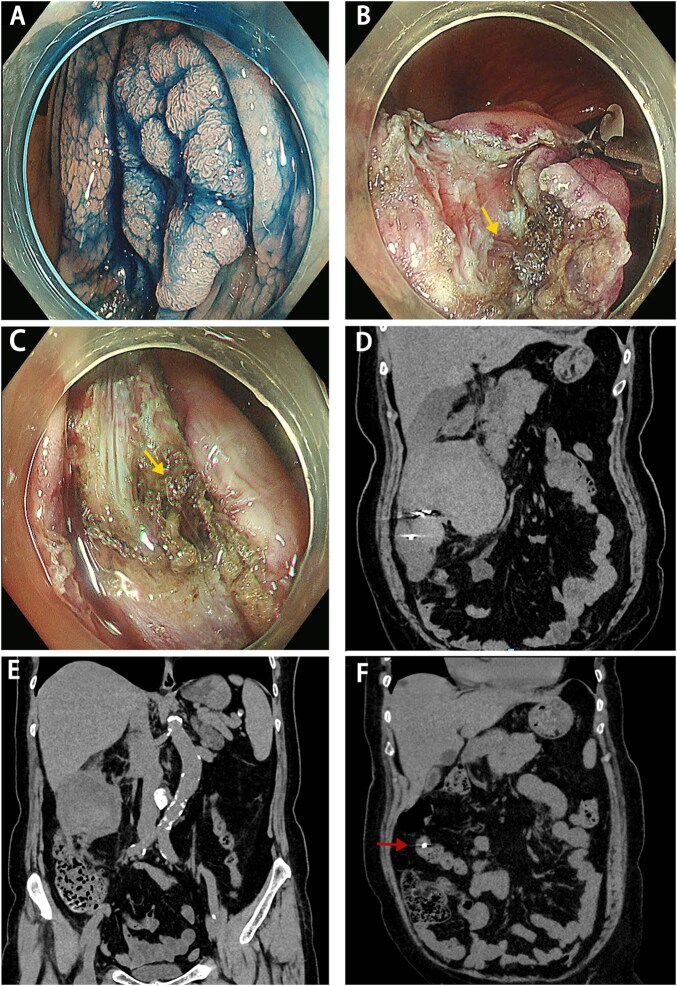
The clinical data of the case with paracolic gutter hematoma after colonic endoscopic submucosal dissection. (A) Indigo carmine chromoendoscopy demonstrated a lateral spreading tumor ∼3 cm in size in the ascending colon. (B) Several perforating vessels originating from the muscularis propria were identified within the central portion of the lesion; the arrow indicates the vessels. (C) After resection, visible vessel stumps could be observed on the ulcer bed, indicated by the arrow. (D) Abdominal CT scan after endoscopic submucosal dissection showed a hematoma in the right paracolic gutter, measuring 11.0 × 6.7 × 11.1 cm^3^; the hyper-dense focus corresponded to the clips at the resection site. (E) One-month follow-up abdominal CT showed a reduction in the size of the right paracolic gutter hematoma to 8.3 × 5.1 × 6.3 cm^3^. (F) Five-month follow-up CT imaging demonstrated complete resolution of the hematoma; the arrow indicates the clips at the resection site.
